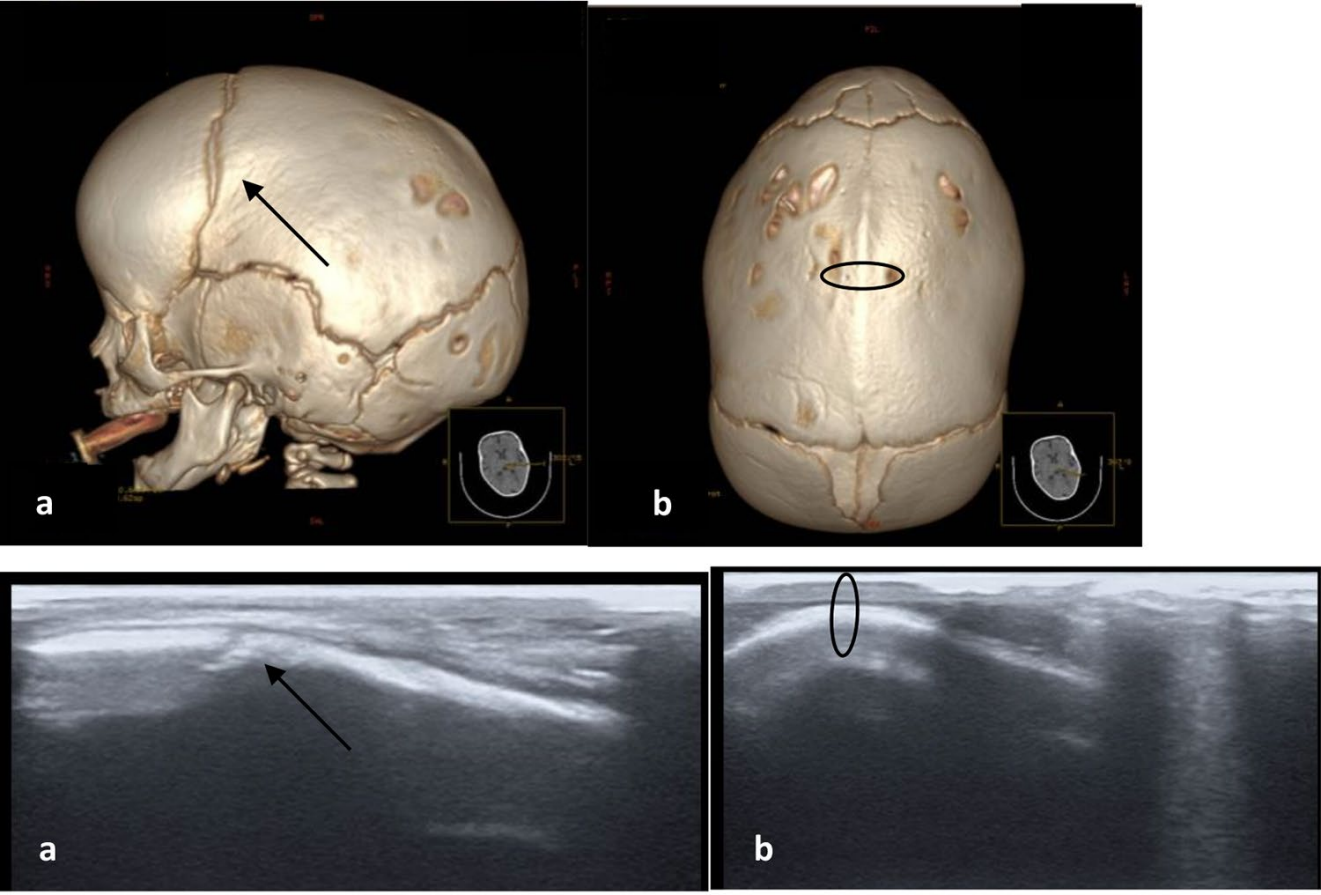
Scaphocephaly. a 3D-CT lateral view: skull shape with elongated cranium, with patent coronal suture (arrow); b 3D-CT superior view: showing total sagittal synostosis (ring), with corresponding US images
